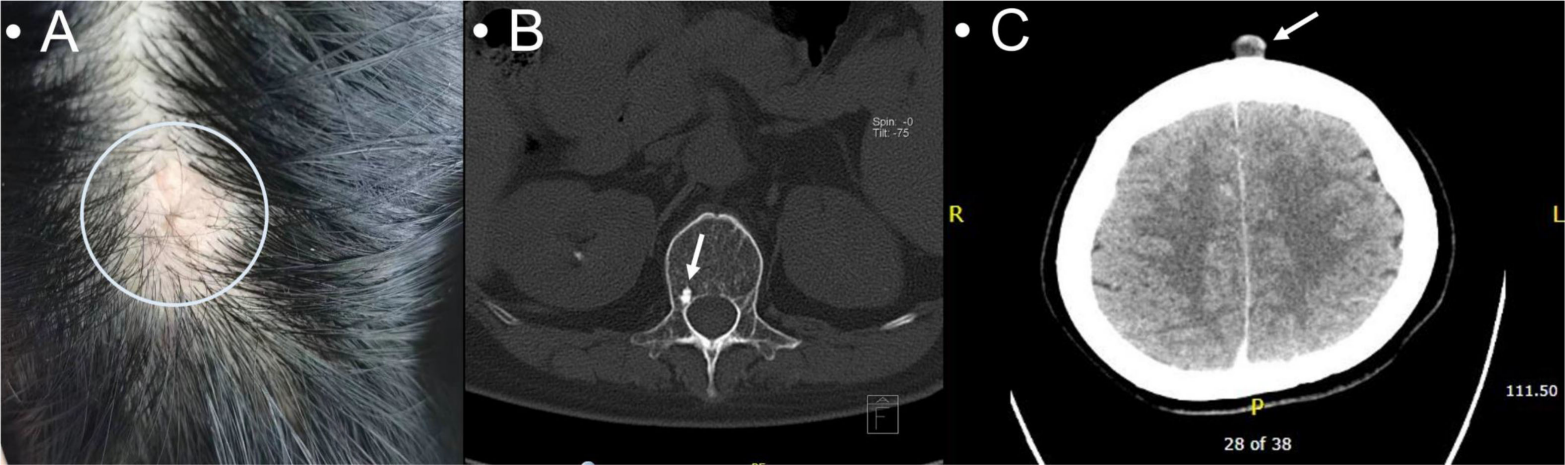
A 2.0 × 0.5-cm non-mobile non-erythematous elevated poorly demarcated nodule in the occipital scalp (A); hyperdensity in the 1st lumbar spine suspicious for metastases (B); a cutaneous occipital scalp nodule on the CT scan (C).
